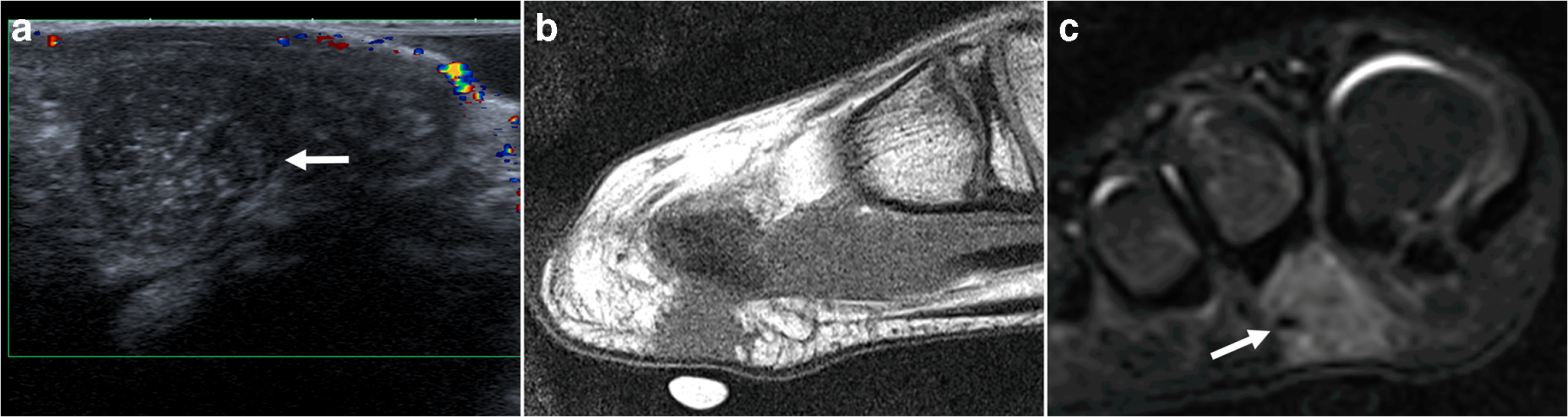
A 13-year-old boy with calcifying aponeurotic fibroma. a Ultrasound image in the sagittal plane shows a well-defined hypoechoic mass (*arrow*) with multiple fine hyperechoic reflections (representing fine calcifications) at the plantar side of the right forefoot. b Sagittal T1-weighted image demonstrates the mass (near the skin marker) to be isointense to muscle. c Coronal turbo inversion recovery magnitude image demonstrates the mass (*arrow*) to be hyperintense. Pathological analysis after resection confirmed aponeurotic fibroma with presence of multiple fine calcifications
